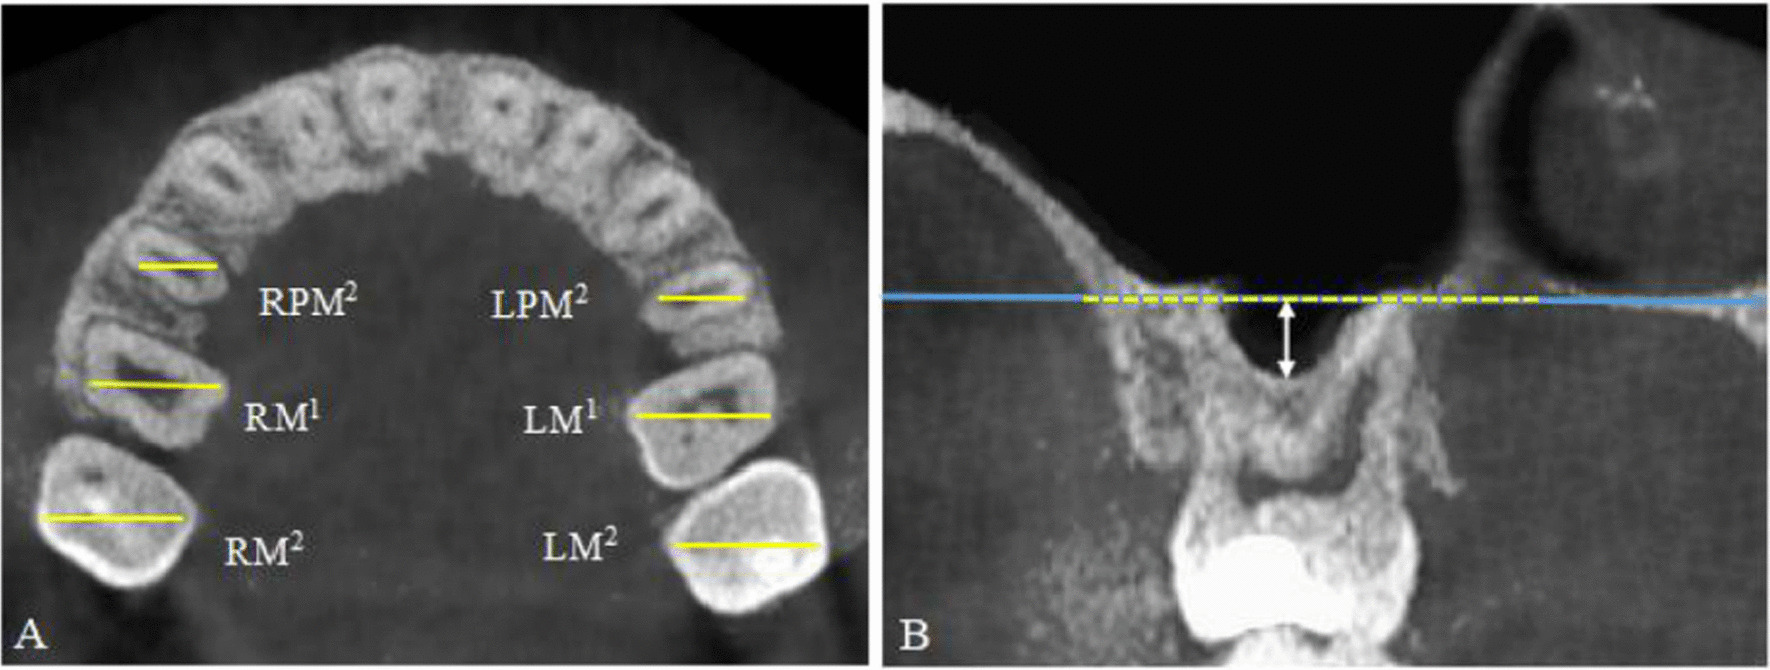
CBCT scanning area and measurement of maxillary sinus pneumatization. A CBCT scanning area: the central area of maxillary second premolars (LPM^2^, RPM^2^), first molars (LM^1^, RM^1^), and second molars (LM^2^, RM^2^) located at the level of the cervical region of the teeth; B Measurement of maxillary sinus pneumatization at RPM^2^ in a female patient: the yellow dashed line refers to the tangent of the nasal cavity floor, and the white double-headed arrow represents the linear distance from the nasal floor to the lowest level of the maxillary sinus floor
